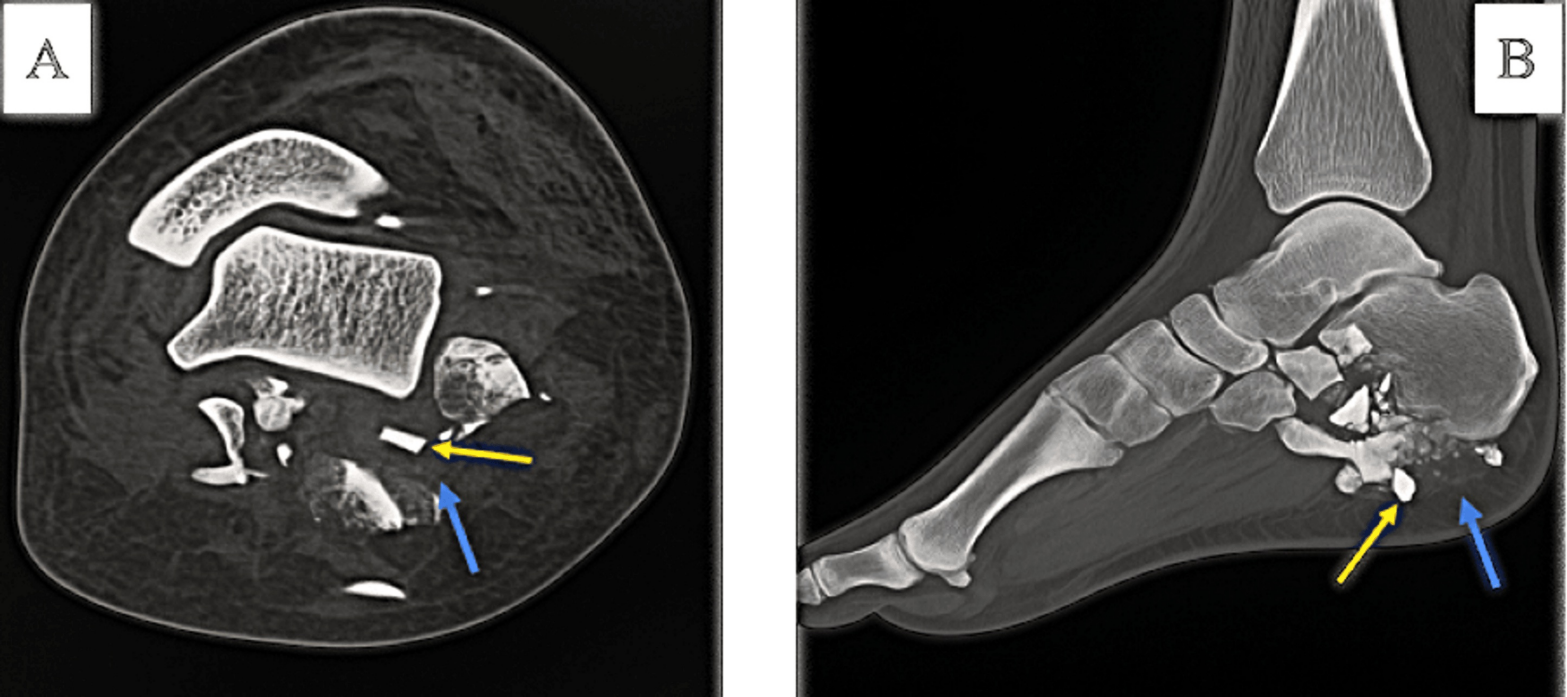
CT scan of the foot transversal view (A) and lateral view (B) with 3D reconstruction showing bone fragmentation of the calcaneus, reduced to small fragments with the destruction of the various intertarsal joint surfaces (talocalcaneal, calcaneo-navicular, and calcaneo-cuboid) (blue arrow) associated to small metallic foreign bodies within the bone fragments (blasting cap) (yellow arrow).
